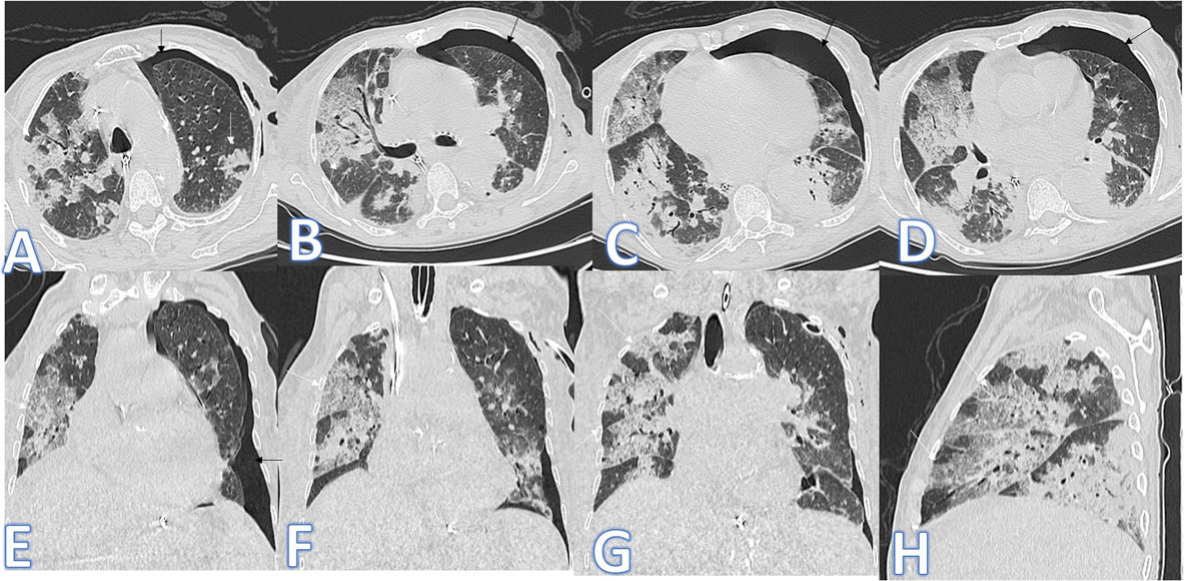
A 72-year-old female patient presented with severe dyspnea with desaturation. She was intubated. CT chest revealed bilateral symmetric confluent patches of ground glass with crazy paving appearance mixed with consolidative patches with air bronchogram more in the lower lobes (white arrows) more on the right side. LT side mild pneumothorax was noted (black arrows). It was diagnosed as AIP after transbronchial biopsy
